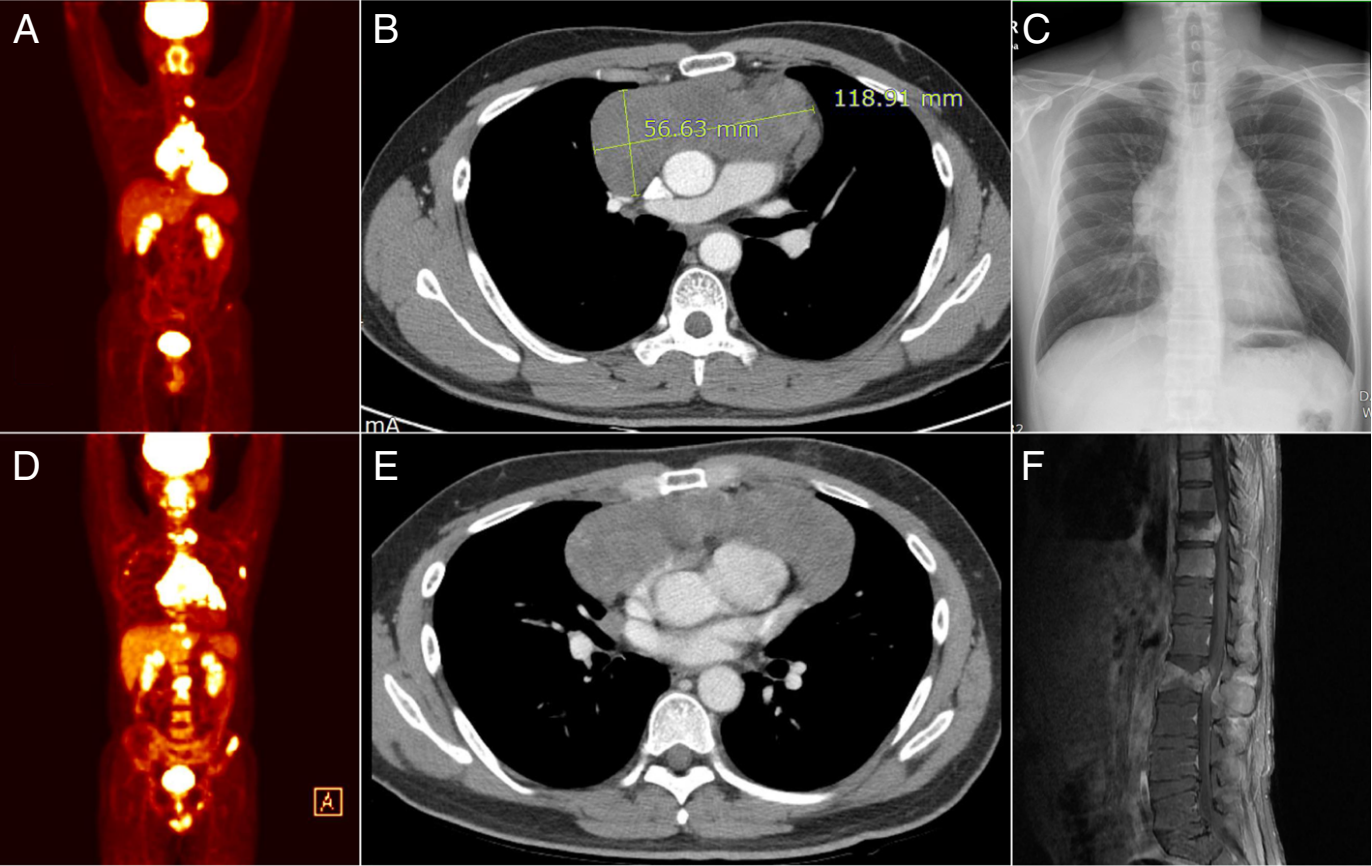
Radiologic presentation of thymic adenocarcinoma and systemic metastases. Top images a, b, and c represent the initial presentation of thymic adenocarcinoma. Bottom images d, e, and f indicate disease progression observed 3 to 5 months after the initial workup. a PET-CT scan shows the large mass in the anterior mediastinum with metastatic lesions. b Chest CT shows 118.91 × 56.63 mm sized mass with focal calcification. c Chest X-ray shows the anterior mediastinal mass. d PET-CT scan after 3 months reveals metastatic lesions to mediastinal lymph nodes and multiple bones. e Chest CT after 4 months reveals the enlarged mass and metastatic lung nodules. f Spine MRI after 5 months reveals the significant cord compression at T10 and severe central canal stenosis at L2
